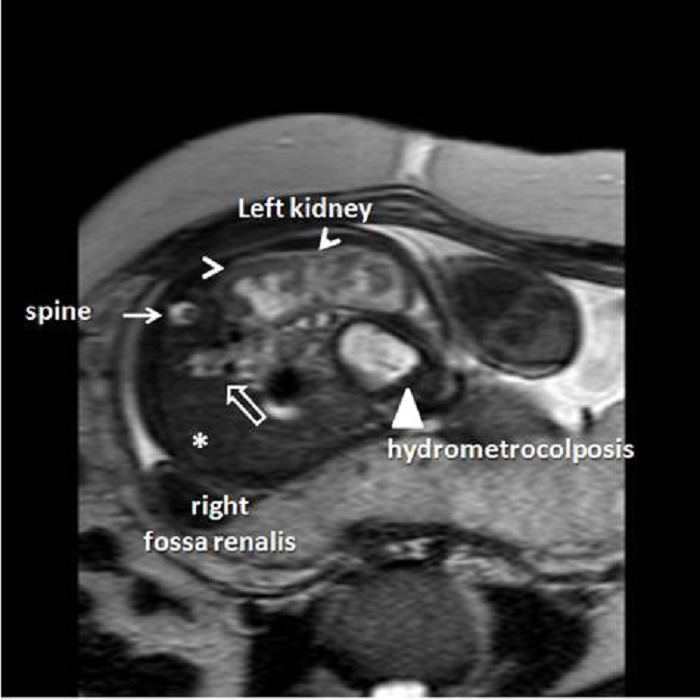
Figure 2B: Left hydronephrotic kidney (white arrow heads) was demonstrated in fetal MRI while right fossa renalis (white asterix) was empty. However, just behind the spine, a structure with a similar appearance to a dysplastic, slightly distorted right kidney was reported (white empty arrow). Hydrometrocolposis was clearly visualized by fetal MRI (white triangle).
